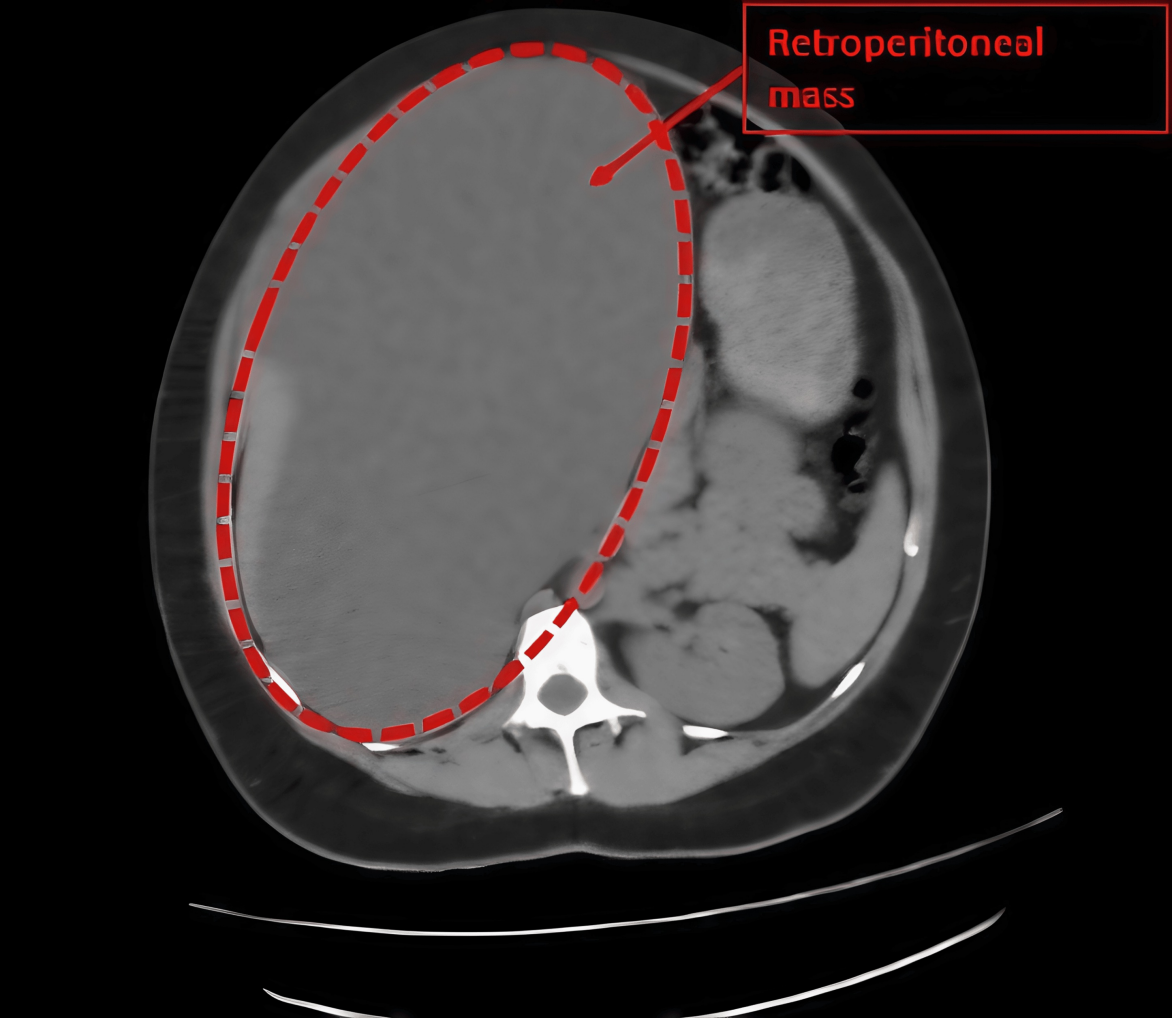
Axial CT scan demonstrating a large retroperitoneal cystic lesion Axial non-contrast abdominal CT image showing a large, well-defined hypodense cystic mass located in the right retroperitoneal space (outlined with a red dashed line). The lesion causes medial displacement of the right kidney and exerts mass effect on adjacent abdominal structures. No internal septations, mural nodules, or calcifications are observed. These imaging characteristics are suggestive of a retroperitoneal cystic lesion. CT: computed tomography
